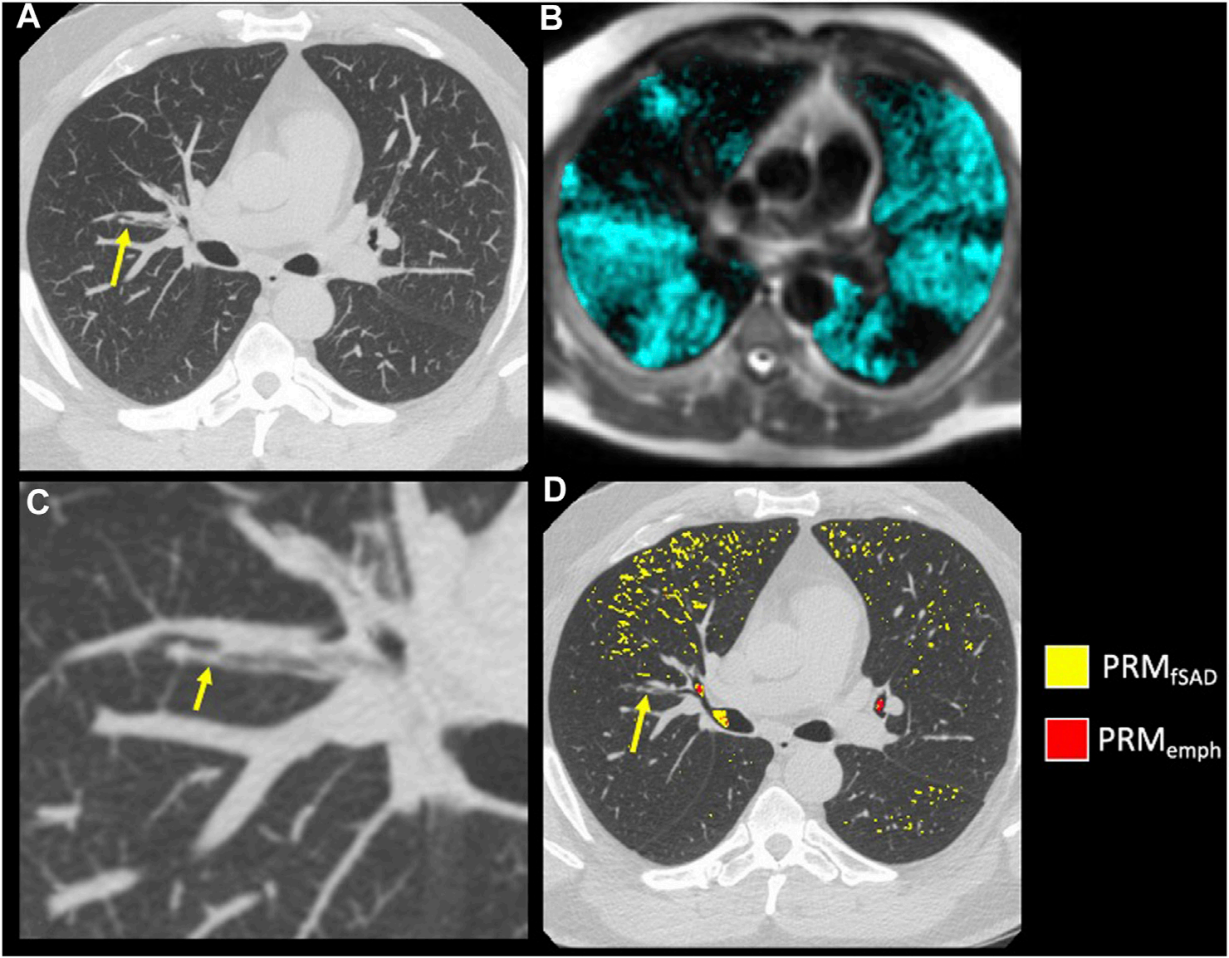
Example of mucus plugging within a moderate asthmatic. (A) A mucus plug (arrow) visualized on inspiratory CT using a MIP of 7 slices. (B) A close-up of the mucus plug (arrow) in (A) (C) HP 3He MRI overlaid on conventional MRI with ventilation defects occurring near and downstream of the mucus plug visualized in (A). (D) PRM maps overlaid on Inspiratory CT in the axial slice of the mucus plug (arrow).
